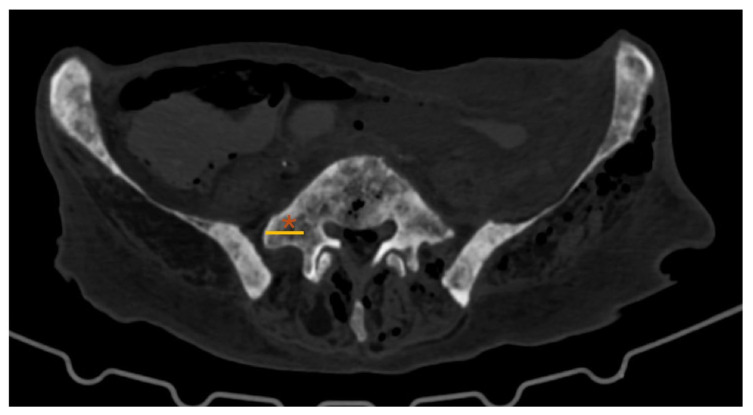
Lumbar spine CT—axial plane; *: L5 transverse process (TP); yellow line: L5 TP length.
